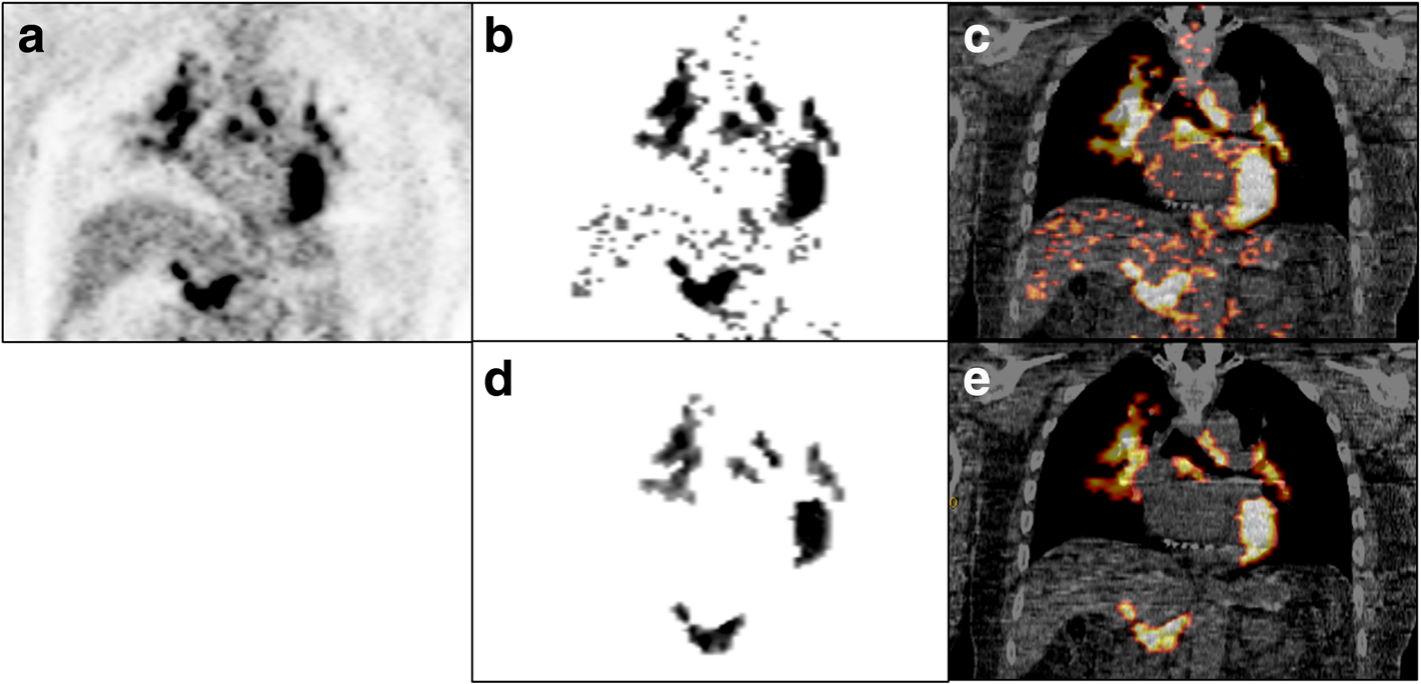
Post-processing of FDG PET/CT images. Attenuation-corrected coronal PET image (a), post segmented PET (using a threshold of max SUV of 2.5) (b) and fused PET/CT (c) images, and post refined PET (d) and fused PET/CT (e) images
